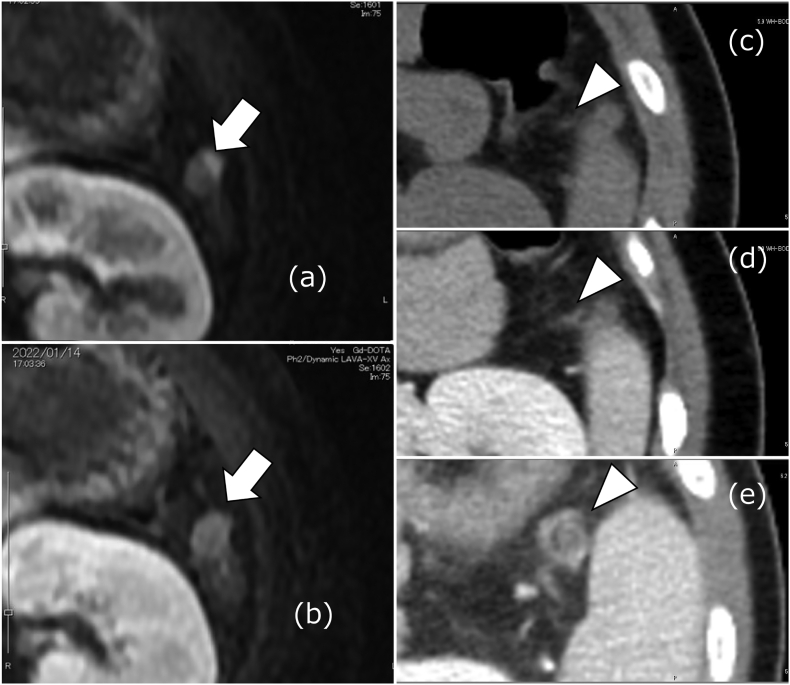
Computed tomography (CT) and magnetic resonance imaging (MRI) at 60 days from the first visit. (a, b) Gadolinium magnetic resonance imaging showed a contrast effect on a part of the accessory spleen and findings of restored blood flow to the accessory spleen (arrows). (c, d) Computed tomography scans showed that the whirlpool sign (arrowheads), observed two months earlier (e; arrowhead), had disappeared.
